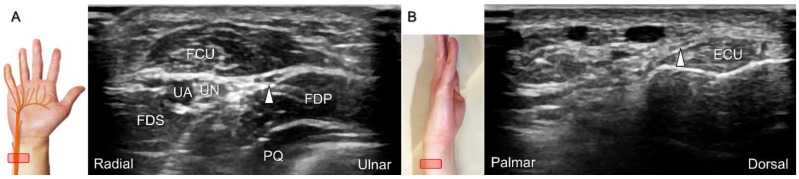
Sonographic imaging of the dorsal ulnar cutaneous nerve as it branches from the ulnar aspect of the ulnar nerve underneath the flexor carpi ulnaris (FCU) muscle (A). The nerve wraps around the distal ulna to reach the dorsal wrist (B). Arrowhead: dorsal ulnar cutaneous nerve. UN: ulnar nerve; UA: ulnar artery; FDS: flexor digitorum superficialis muscle; FDP: flexor digitorum profundus muscle; PQ; pronator quadratus muscle; ECU: extensor carpi ulnaris tendon.
